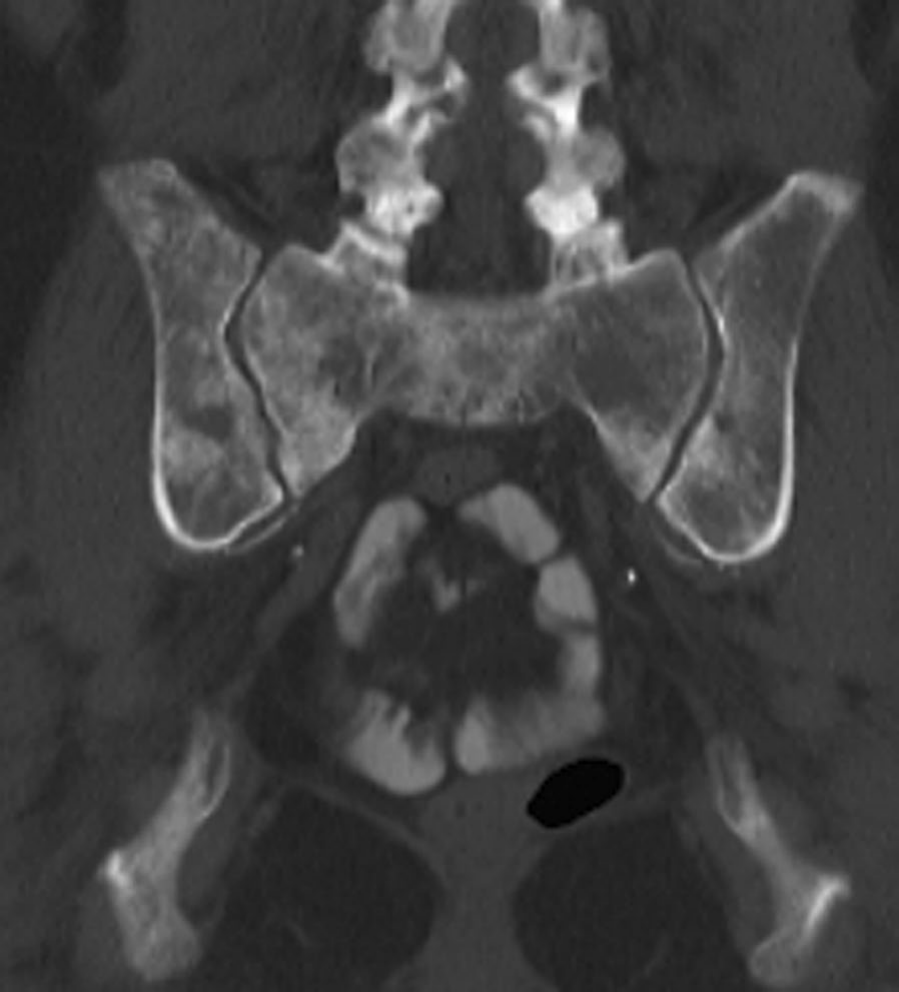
CT scan of the pelvis. Multiple osseous metastases of pelvis were detected
